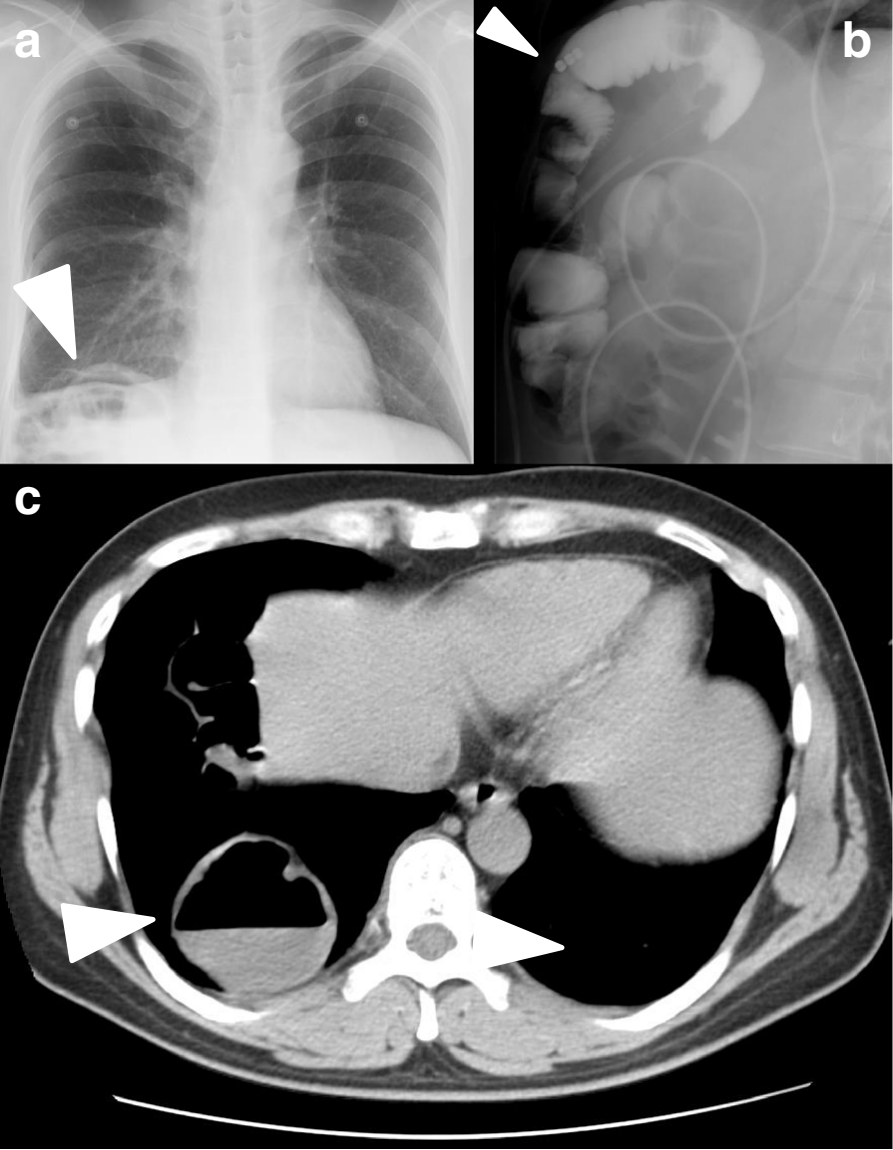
Preoperative examinations. Chest and abdominal plain radiographic findings. a The chest radiograph revealed intestinal air in the right lower lung field (arrow head). b The abdominal radiograph revealed dilation of the large intestine with gas (arrow head). c Computed tomography showing the herniated transverse colon located in the right thoracic cavity (arrow head)
